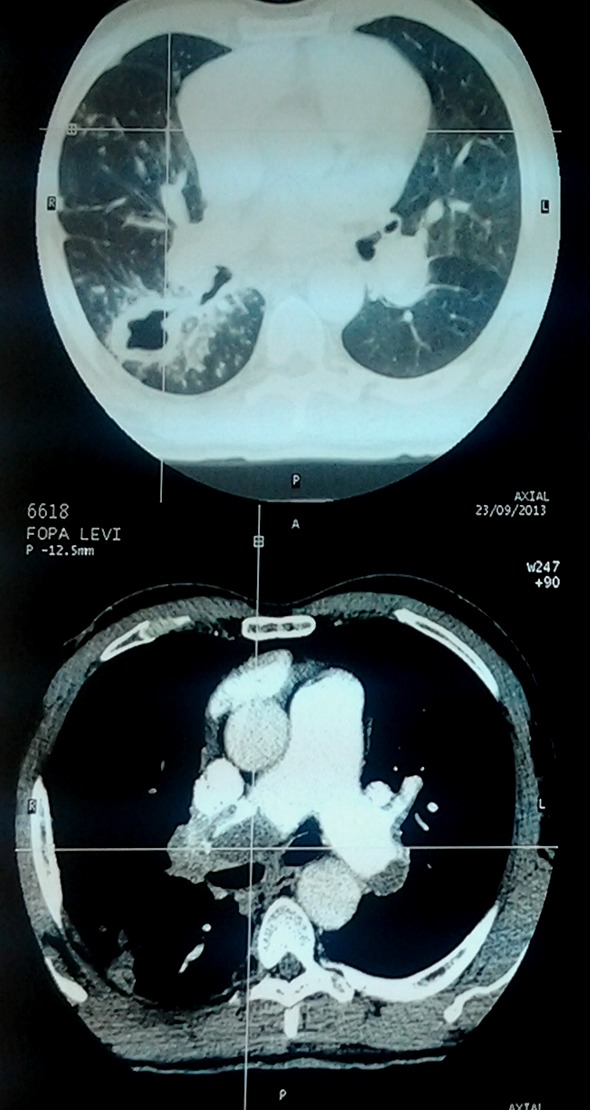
Thoracic CT scan showing cavitation in upper lobe of the right lung and bilateral pulmonary embolism
